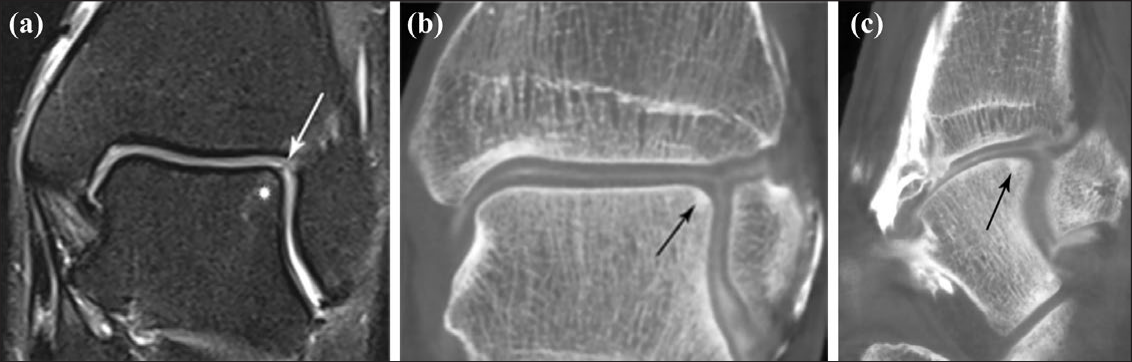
Down staging of an OCL on CBCT compared to MRI. Coronal (a) fat suppressed T2-WI show adjacent BME (white asterisk) at the superolateral aspect of the talar dome. The overlying cartilage is difficult to assess on MRI but seems to be slightly inhomogeneous (white arrow). Coronal (b) and sagittal (c) reformatted CBCT-A show subtle subchondral sclerosis (black arrow) at the superolateral aspect of the talar dome, but the overlying cartilage is intact.
